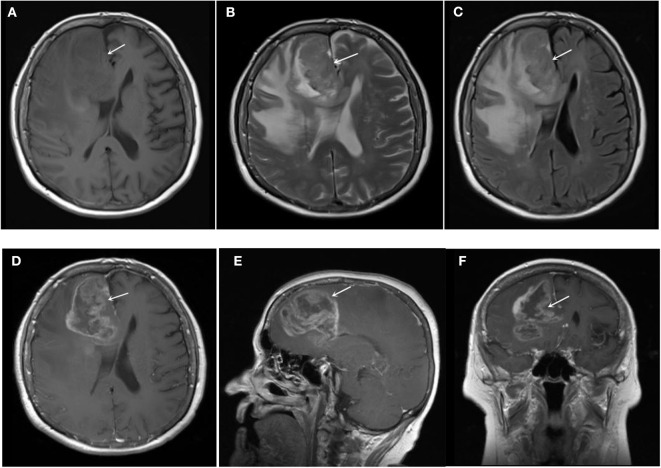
IDH1 wild-type glioma (low grade): (A) T1WI (B) T2WI (C) FLAIR (D) T1+C (axial) (E) T1+C (sagittal) (F) T1+C (coronal). The lesion had unclear border, heterogeneous signal intensities on T1WI, T2WI, and FLAIR. The lesion presented markedly heterogeneous enhancement on post-contrast T1WI.
